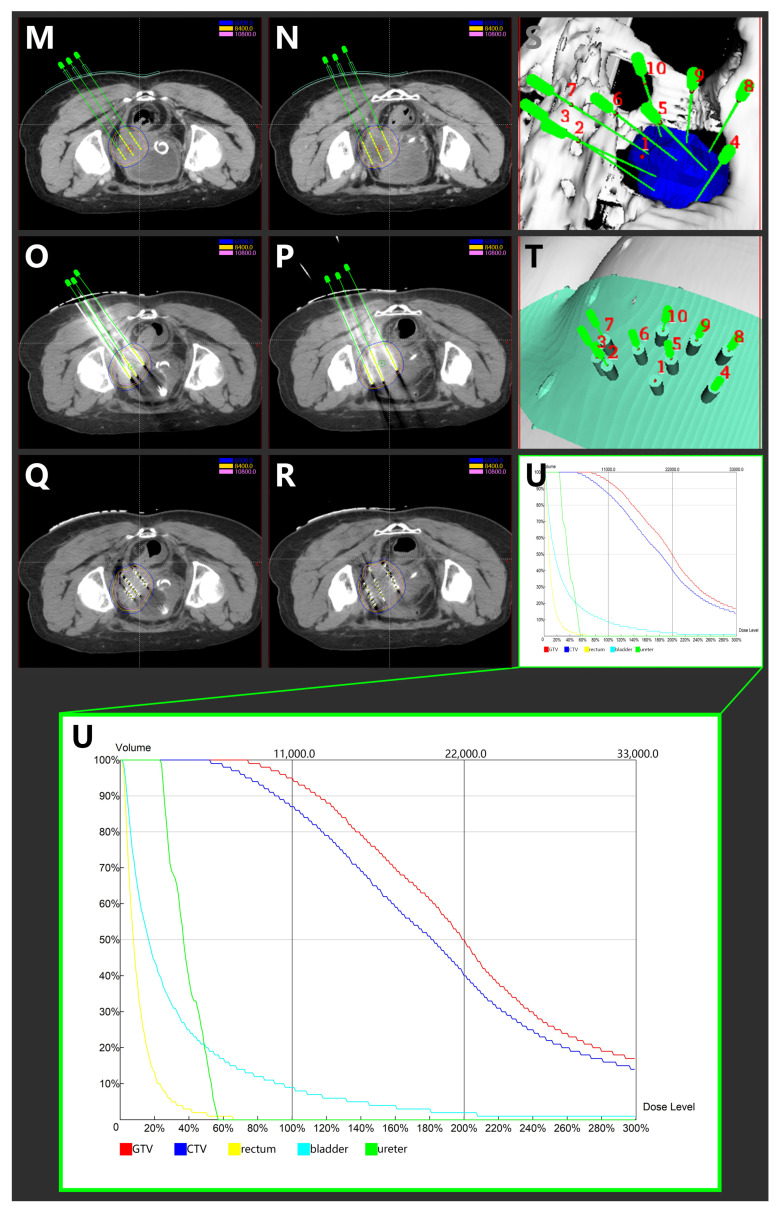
(A,B) Magnetic resonance imaging taken before CCRT in T2-weighted sequence. (C,D) Magnetic resonance imaging taken after CCRT and ACT in T2-weighted sequence. (E,F) Magnetic resonance imaging depicting the cervical cancer recurrence after CCRT and ACT in T2-weighted sequence. (G,H) Magnetic resonance imaging depicting the cervical cancer recurrence after CCRT, ACT, and HDR-IIB in T2-weighted sequence. (I,J): Magnetic resonance imaging taken after 3D-PNCT assisted RISI in T2-weighted sequence. (K,L) Magnetic resonance imaging taken after 3D-PNCT assisted RISI in T2-weighted sequence. (CCRT, concurrent chemoradiotherapy; ACT, adjuvant chemotherapy; HDR, High-dose-rate; IIB, interstitial implantation brachytherapy; 3D, 3-dimensional; PNCT, printing non-coplanar templates; RISI, radioactive 125I seed implantation). (M,N) Portion of pre-plan images. (O,P) Portion of real-time dose optimization images. (Q,R) Portion of post-plan images. (S,T) 3D-PNCT assisted RISI reconstruction visualization for recurrent cervical cancer. (U) Dose-volume histogram of 125I seeds. (3D, 3-dimensional; PNCT, printing non-coplanar templates; RISI, radioactive 125I seed implantation).
